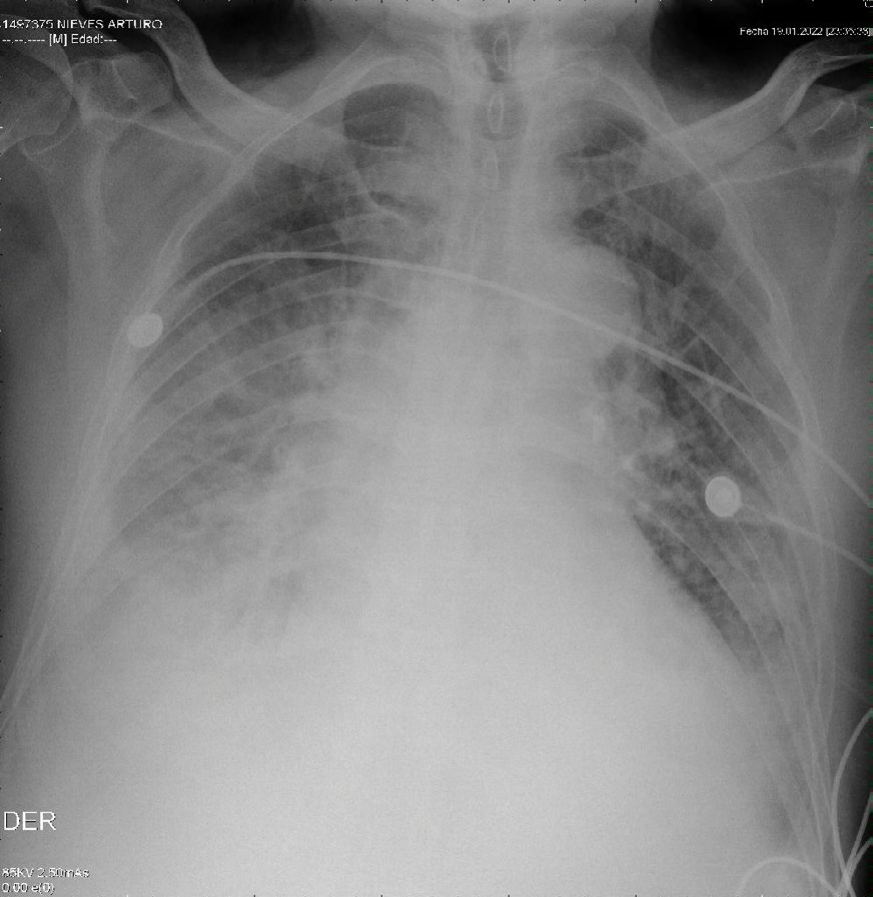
Chest X-ray upon admission (01/13/2023)
